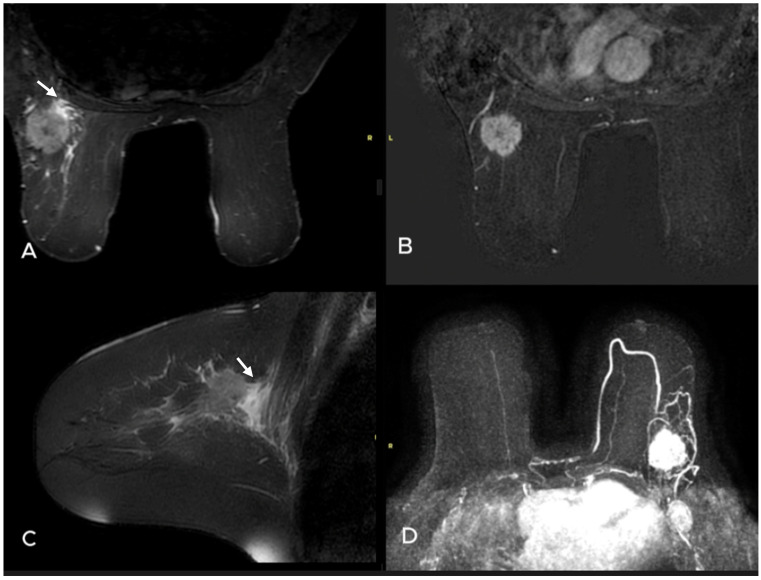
A 49-year-old woman presented with a palpable mass in the left breast. T2W (A) and subtracted dynamic (B) MR images revealed a round mass with irregular margins that showed heterogeneous enhancement. There was prepectoral and subtle perilesional edema on the axial (A) and sagittal (C) STIR TSE images (white arrows). Maximum intensity projection image also demonstrates a pathologic lymph node in the axilla (D). Pathology: Invasive ductal carrcinoma ER: 90%, PR: 50% C-erb B2 score: 0, Ki-67 proliferation index 40%, core-needle biopsy of the axilla: metastasis.
